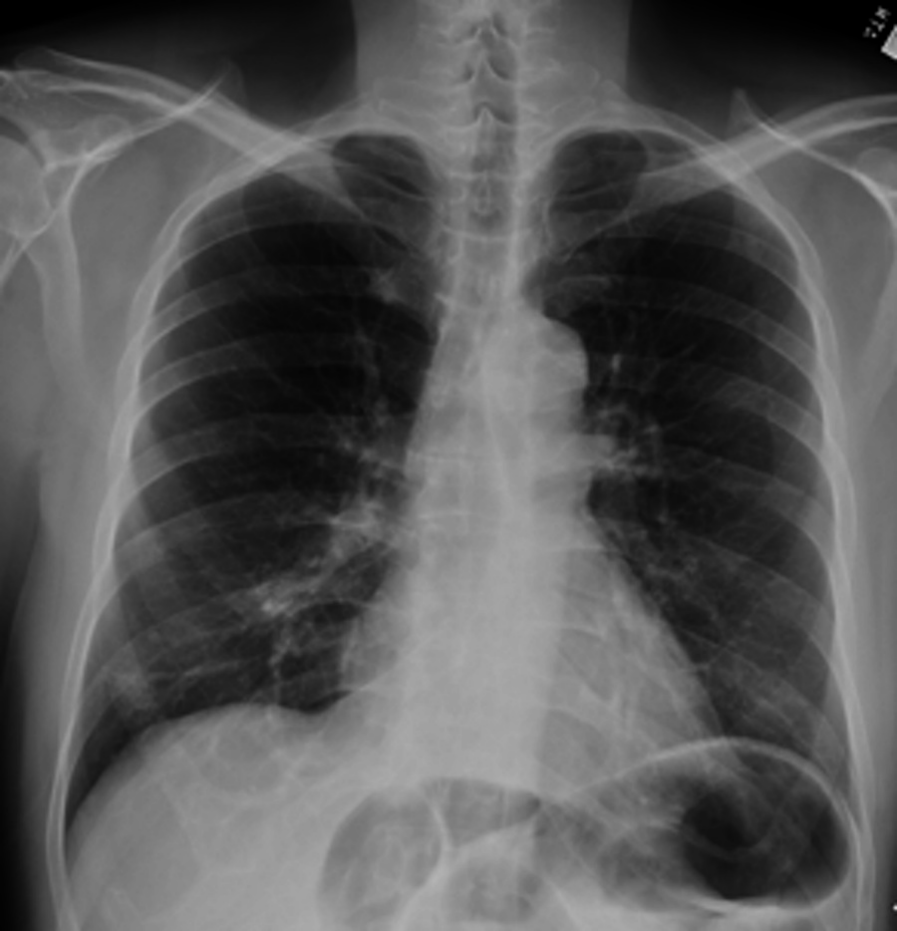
Chest X-ray taken eight months later showed significant resolution of the nodules/masses.
